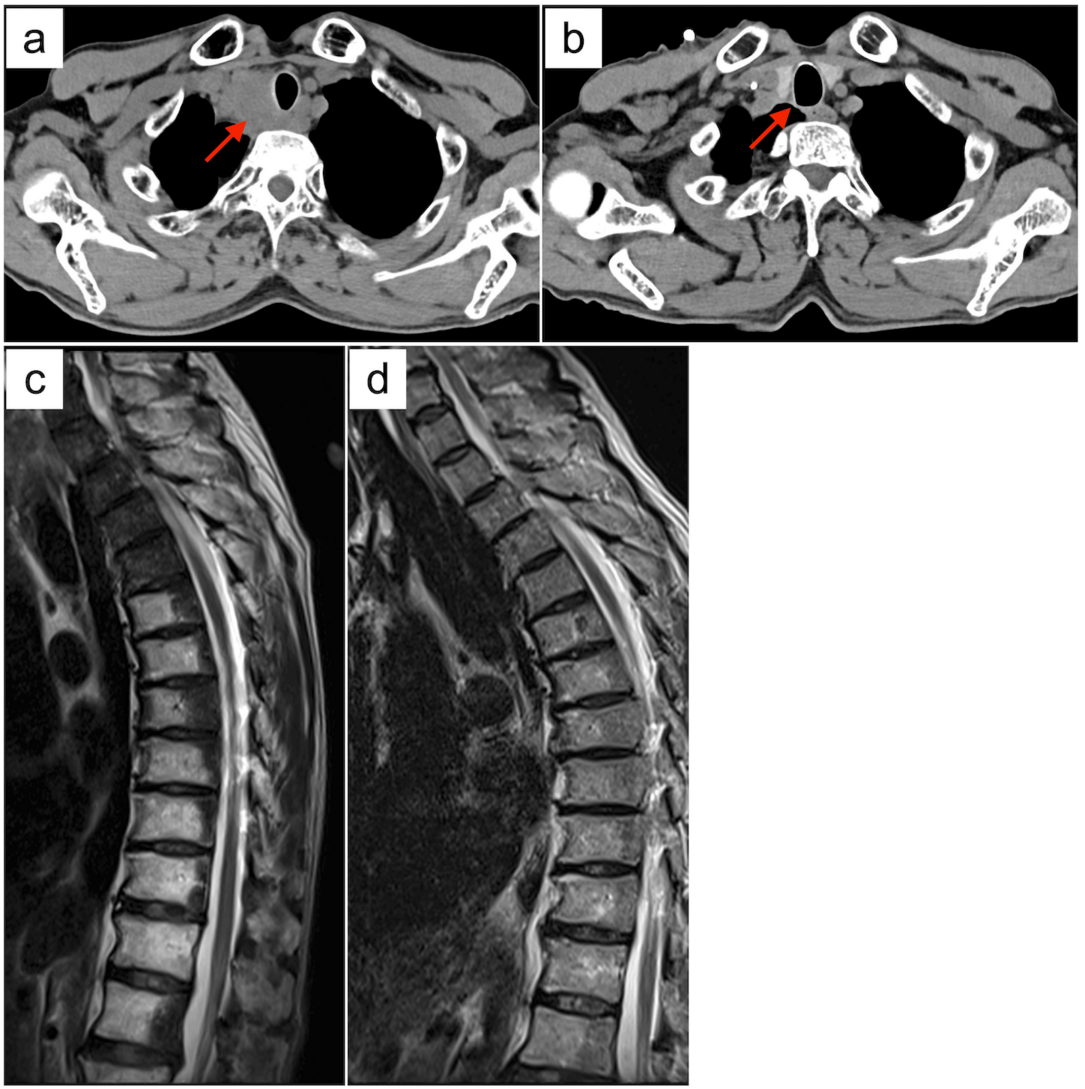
Imaging findings before and after the treatment. Computed tomography shows a mediastinal lymph node metastasis (arrow) directly invading the trachea at baseline (a), which completely disappeared 1 year after ICIs were discontinued (b). Magnetic resonance imaging demonstrates multiple metastases of vertebrae at baseline (c), which were indistinguishable but not totally disappeared 1 year after ICIs were discontinued (d).
